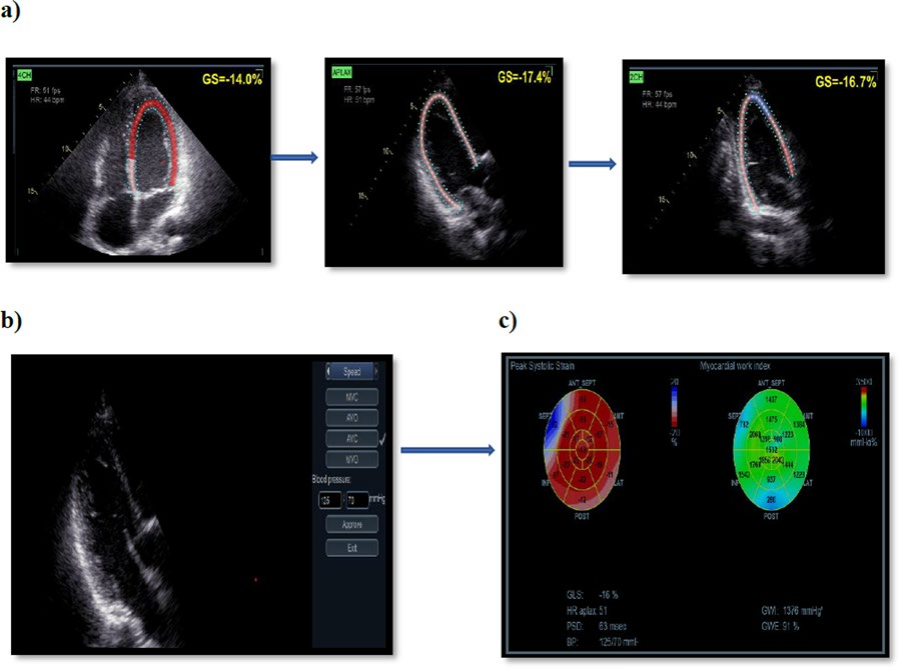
Determination of LV myocardial work. a) the process of measuring GLS from apical 4,3 and 2 chambers. b) systolic and diastolic blood pressure levels have to be entered for LVMW assessment. c) LVMW end results
